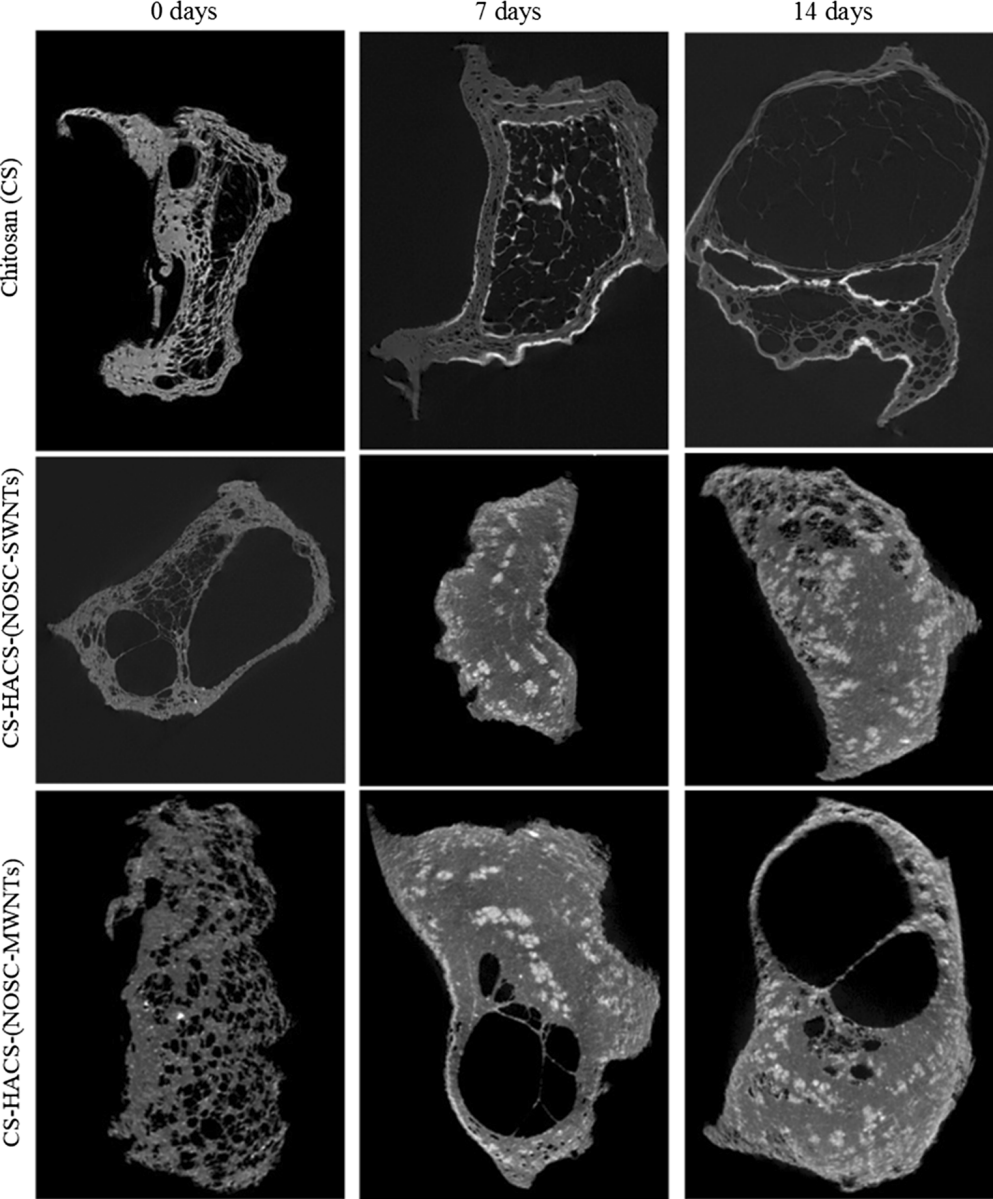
Representative micro CT slices of different gel samples incubated in SBF for 0, 7 and 14 days
